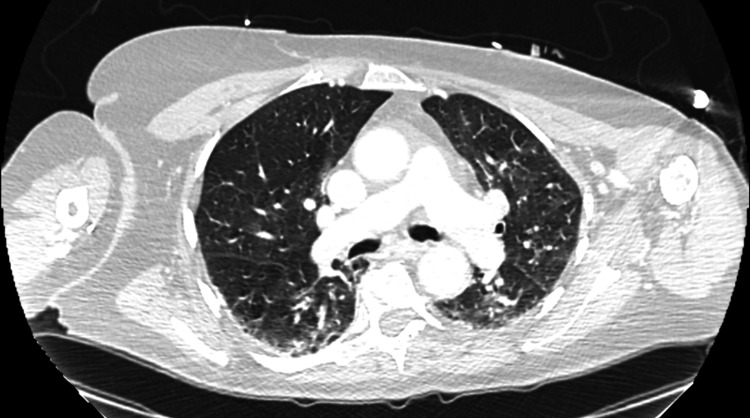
CT of the Thorax (Axial View) Axial view of the CT of the thorax/abdomen/pelvis revealed some prominence of interstitial lung markings, which is nonspecific but can be consistent with pneumonia. CT: computed tomography
